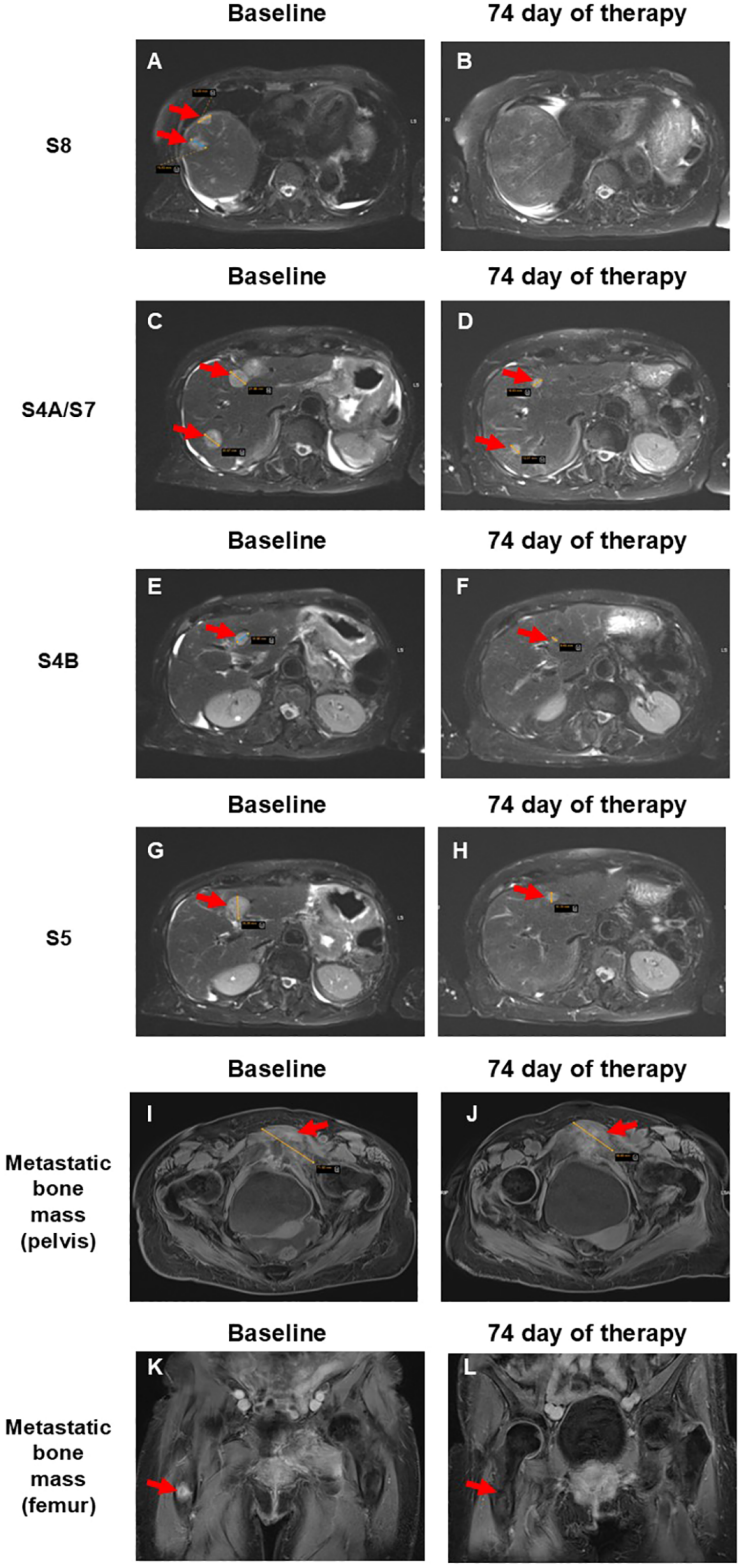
Comparison of pre- and post-therapy MRI scans of a patient with liver and bone metastases from pancreatic cancer after copocaudalic resection following SL-28 therapy. (A, B) Disappearance of two lesions measuring 2.0 and 1.5 cm in the S8 segment. (C, D) Reduction of lesions in the S4A and S7 segments from 2.5 and 2.2 cm to 1.0 and 1.1 cm, respectively. (E, F) Segment 4B lesion decreased from 1.5 cm to 0.5 cm. (G, H) Reduction of the lesion in S5 from 2.3 cm to 1.7 cm. (I, J) Metastatic bone mass near the left pubic bone decreased from 7.2 to 5.7 cm. (K, L) Disappearance of metastatic bone mass in the right femur. Red arrows indicate metastatic lesions.
